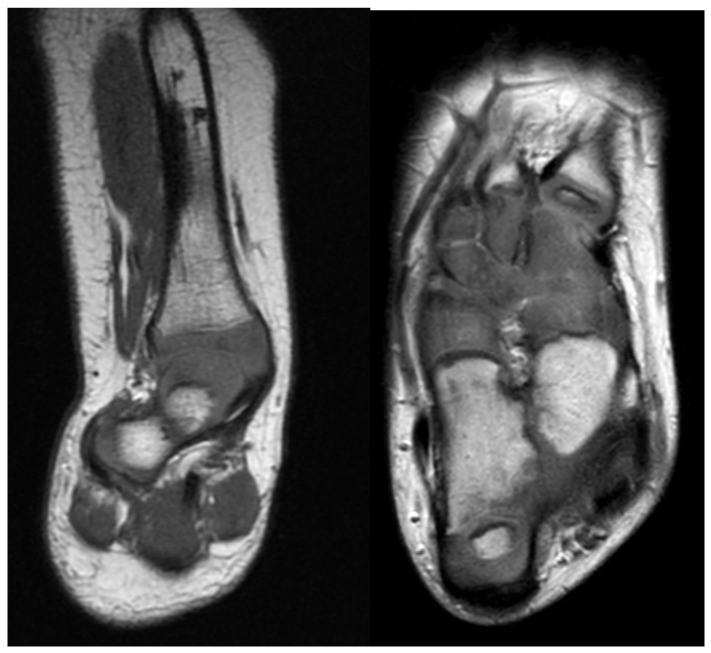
Left is coronal cut of magnetic resonance imaging (MRI) through the ankle and subtalar regions. This shows a subtalar coalition with the Calcaneus to the side of the talus. The ankle has a ball and socket shape. Right is axial cut of MRI through the foot showing the malunited talo-calcaneal coalition with the calcaneus to the side of the talus. The talonavicular and calcaneocuboid coalitions are also seen.
